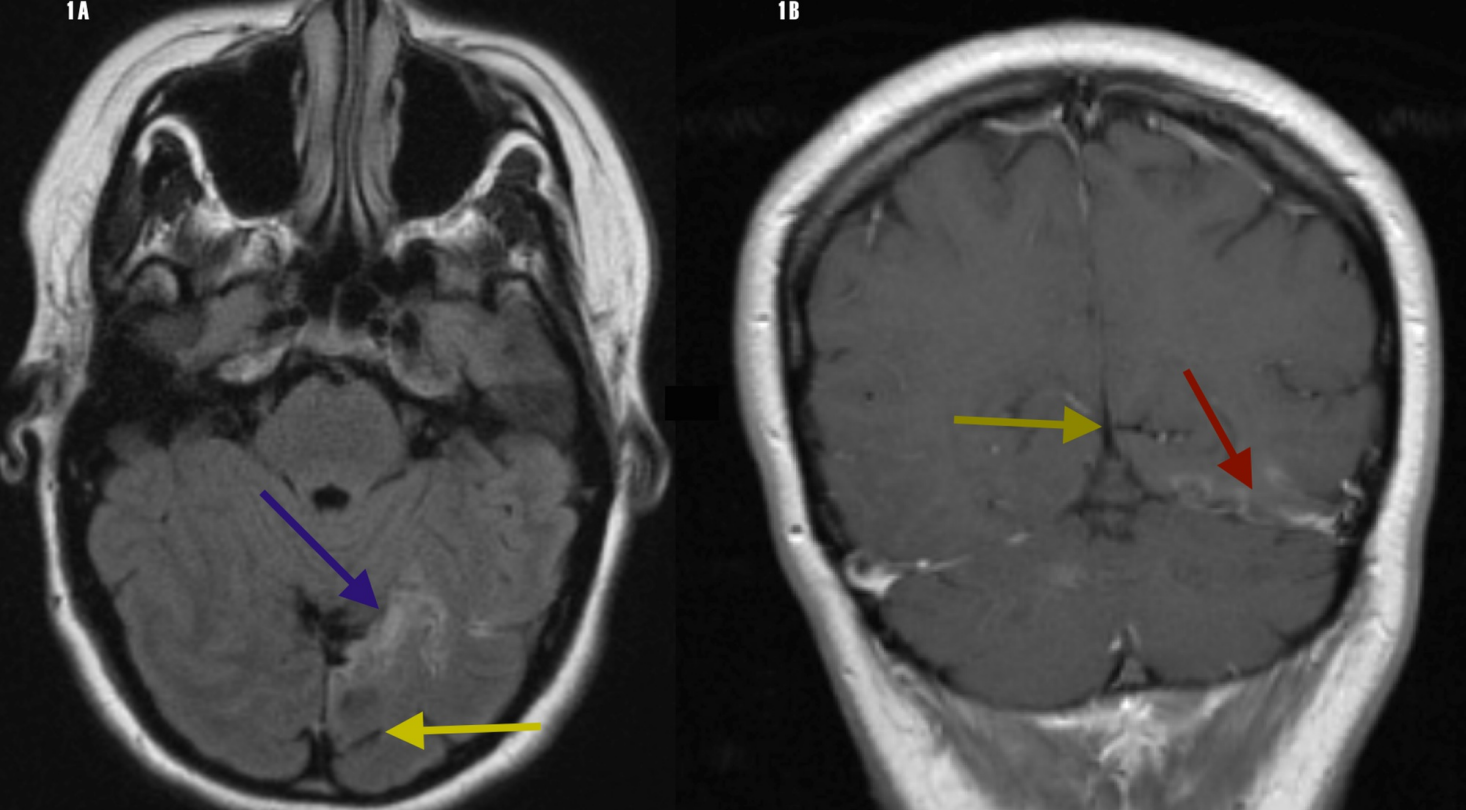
MRI: 1A. FLAIR axial image - left lingual gyrus (blue arrow) and calcarine sulcus (yellow arrow). 1B. T1 gadolinium-enhanced coronal image - left lingual gyrus (red arrow) and calcarine sulcus (yellow arrow) MRI: magnetic resonance imaging; FLAIR: fluid-attenuated inversion recovery; and T1 gadolinium-enhanced image
